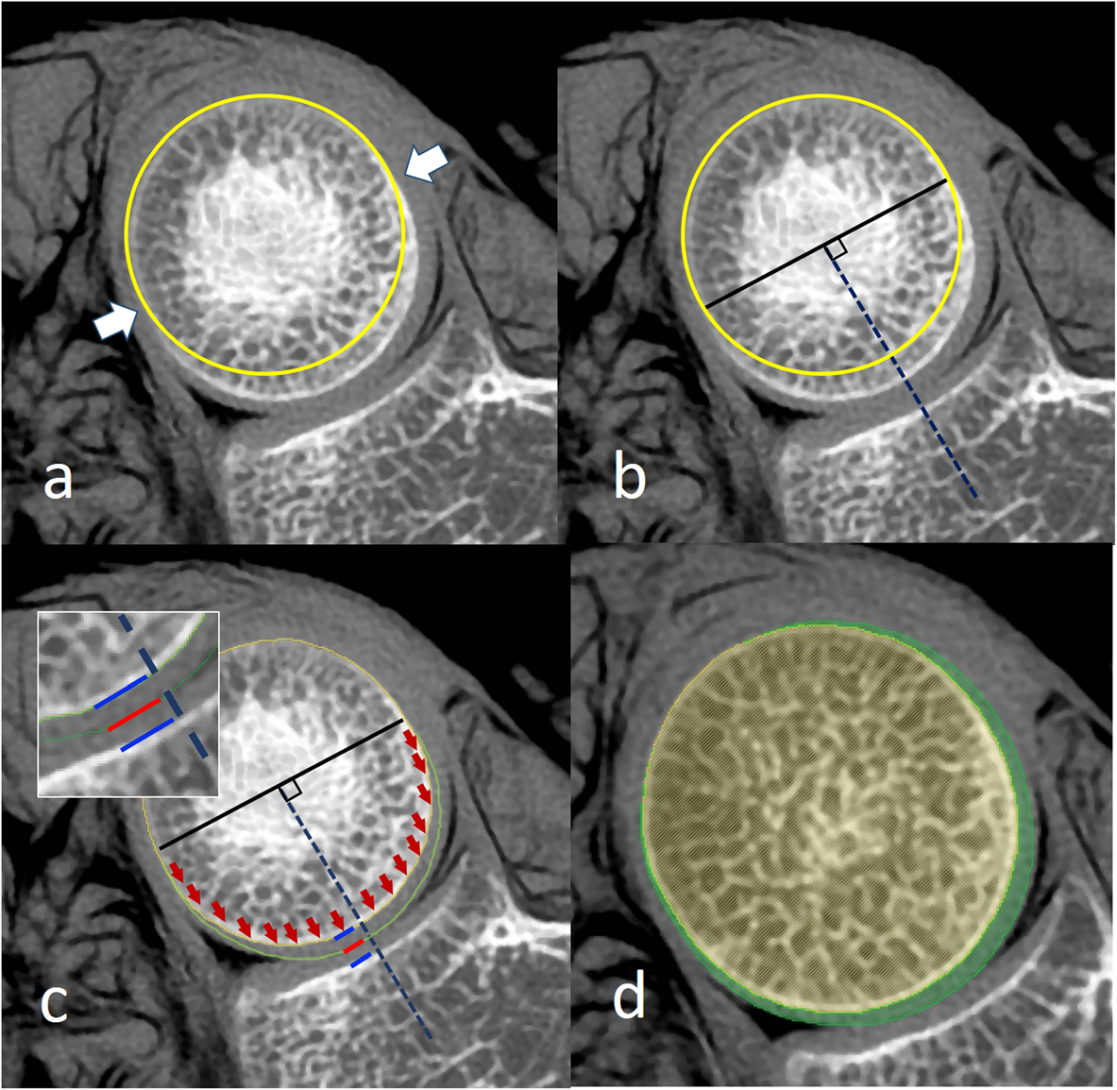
The cartilage-reproducing image reconstruction method of CT image at proximal radioulnar joint. a Determine the ANA junctions (white arrow) using an alteration of the circular curve of nonarticular portion. b Create a reforming axis (blue dash line) using perpendicular line from the midpoint of the straight line between both ANA junctions. c Translate the articular portion along this axis until a reformed border touches half the distance between the subchondral surfaces at the mid portion of radial notch. d Fill the area over ANA junctions until the uneven borders are on the same arch. ANA: articular-nonarticular
